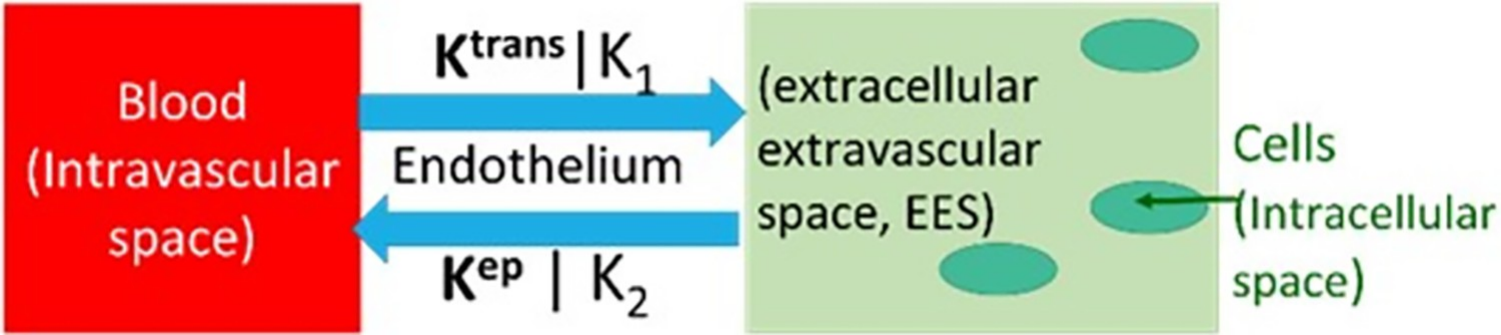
Regional uptake and clearance parameters K_1_ and k_2_ indicated in a one-tissue compartment model implemented in FlowQuant software for ^13^NH_3_ and Toft’s model with transfer constant K^trans^ and K_ep_ used by DB DCE-MRI deconvolution analysis.
